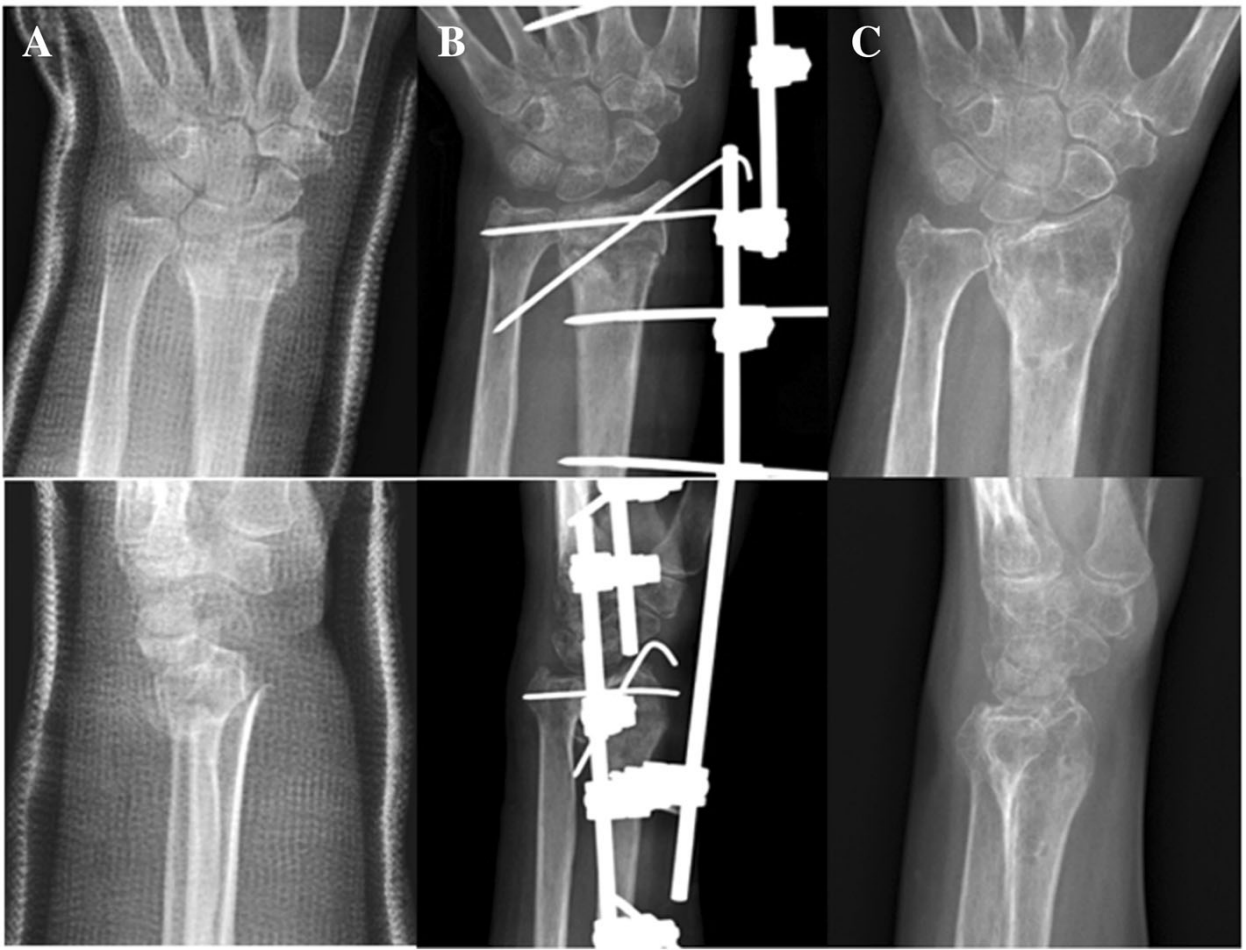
The 67-year-old female with right distal radius fracture (AO type C2) and the ipsilateral radiocephalic shunt was treated with external skeletal fixation and K-wires. The serial images are preoperative (a), immediately postoperative (b), and 1-year follow-up (c) radiographs. The upper row was the anteroposterior view and the lower row was the lateral view
